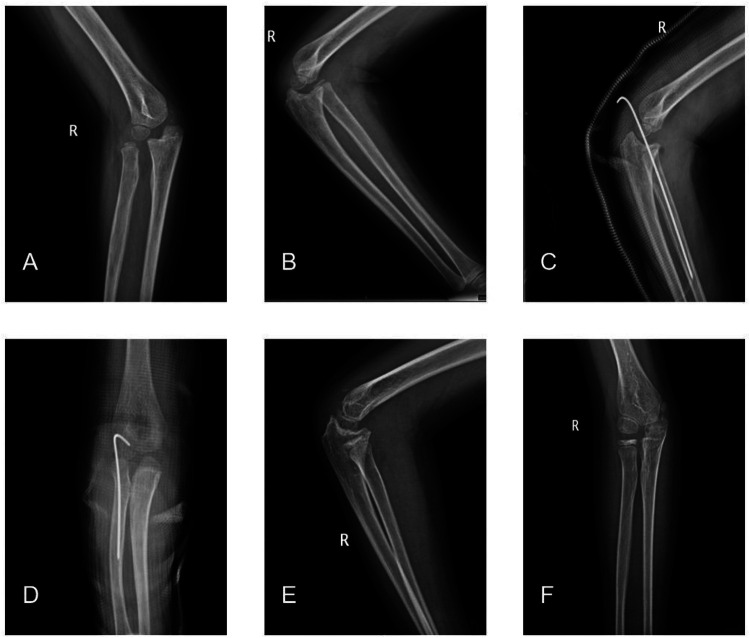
(A,B) Preoperative anteroposterior and lateral images of the right elbow showing an anterior lateral dislocation of the right radial head. (C,D) Anterolateral images of the right elbow on the first day after the open reduction and internal Kirschner wire fixation of the humeroradial joint. (E,F) A 2-year postoperative follow-up x-ray of the right elbow joint, with good alignment of the right humeroradial joint.
